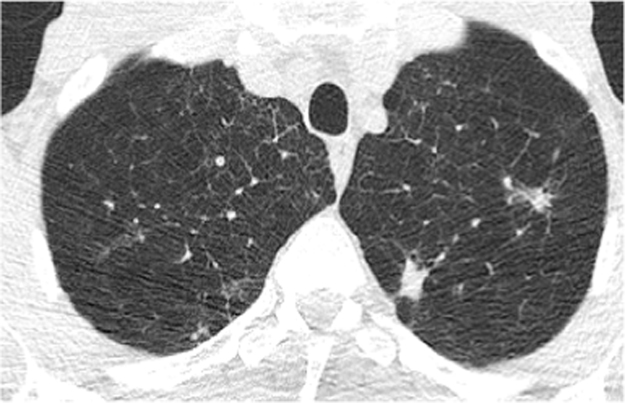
CT thorax from May 2016 demonstrating more prominent nodules.
